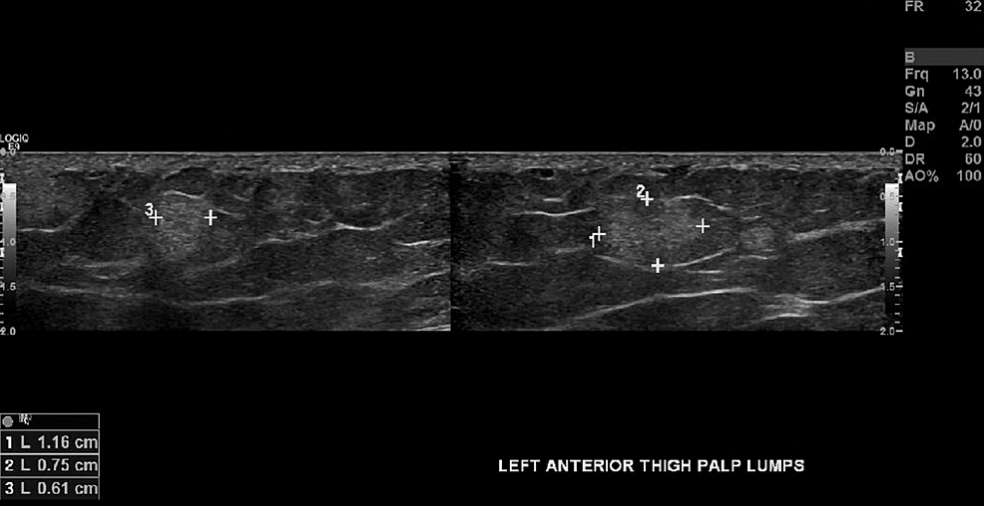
Ultrasound image of a 1.16 x 0.61 x 0.75 cm left anterior thigh soft tissue mass
